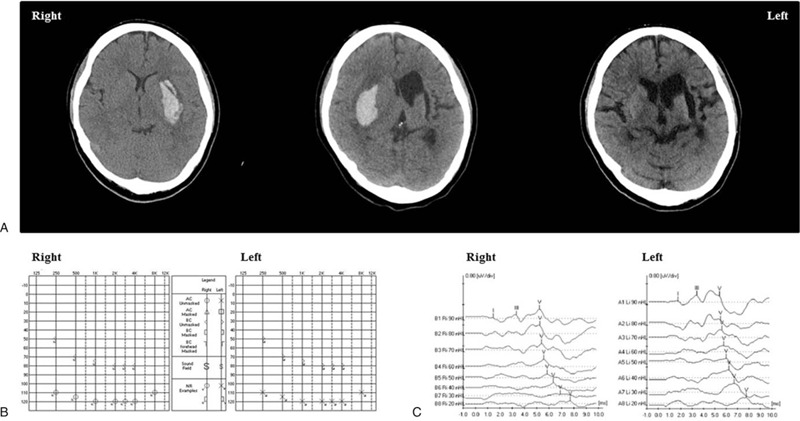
Brain CT were shown in (A) at immediately after the first hemorrhagic stroke (left panel), immediately after the second hemorrhagic stroke (middle panel) and 6 mo after the second hemorrhagic stroke (right panel). The results of pure tone audiometry and brainstem auditory evoked potential test were shown in (B) and (C), respectively.
